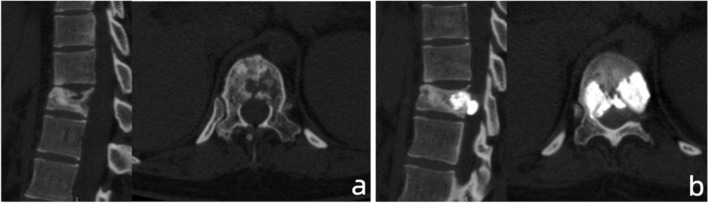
A 27-year-old female patients with breast cancer presented with T11 pathological fracture. a Preoperative CT showed the destruction of posterior wall and mild compression of the vertebral body. b Postoperative CT presented the leakage of cement into the spinal canal
